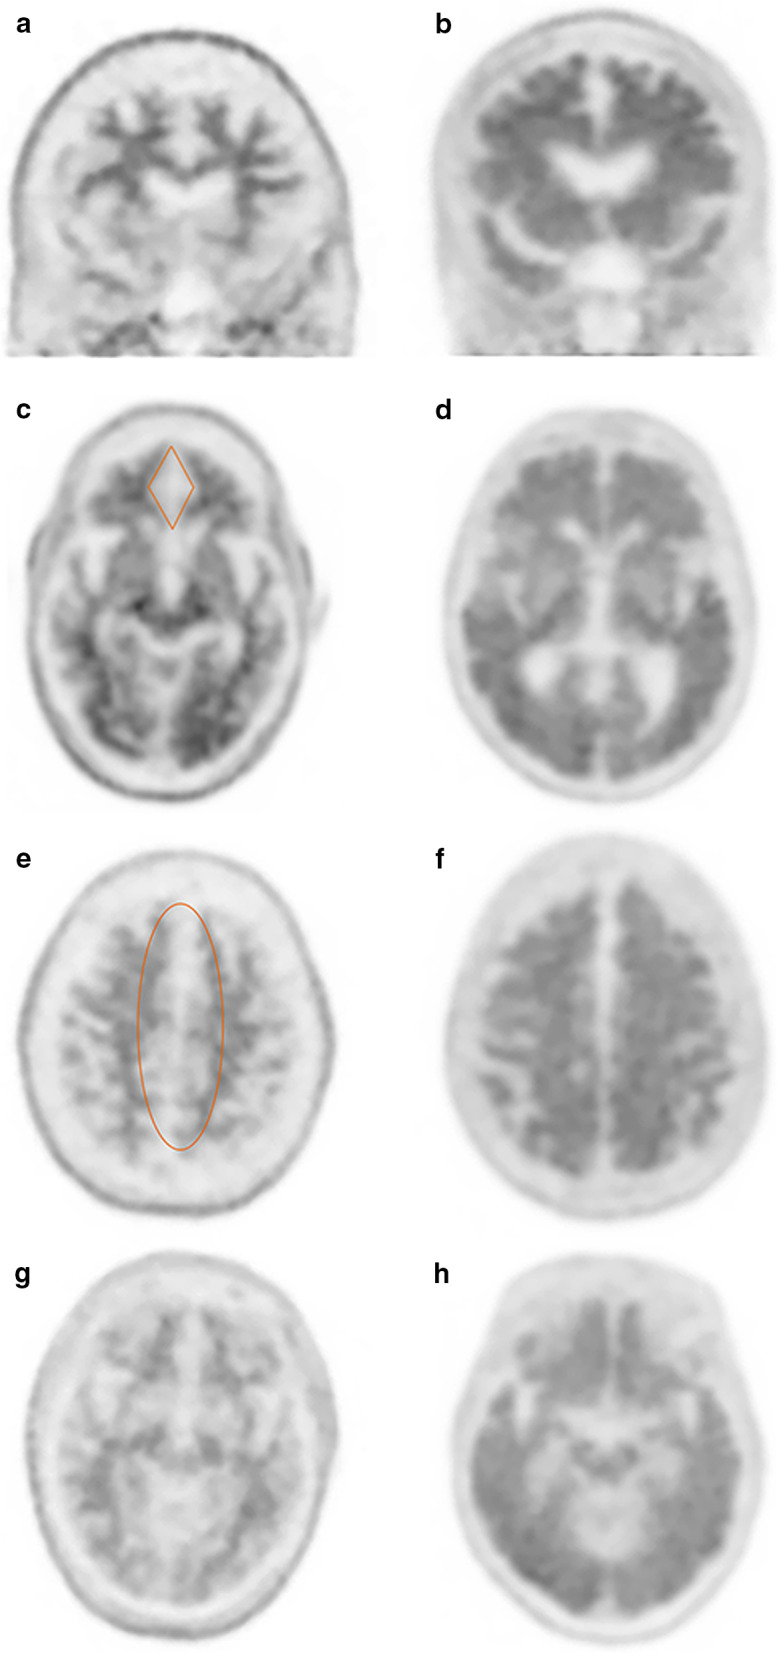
Named signs of normal and abnormal patterns of amyloid activity on florbetapir PET/CT. Normal activity in the white matter of the frontal lobes leads to the “tree-in-winter sign” on coronal images, abnormal activity in the gray matter leads to the appearance of leaves known as the “tree-in-summer sign” (a, b). Absence of gray matter activity in the medial orbitofrontal lobes creates a diamond pattern of the white matter activity which is lost in abnormal studies (c, d). A similar phenomenon is seen at the cerebral convexities where the superior white matter tracts create the “double convex lens sign” (e) which is lost with abnormal gray matter activity morphing to the “kissing hemispheres sign” (f). Lastly, normal absence of gray matter activity in the temporal and occipital lobes creates a mountainous façade referred to as the “temporo-occipital ridge” (g), whereas abnormal gray matter activity leads to a more flat profile termed the “temporo-occipital plain” (h)
